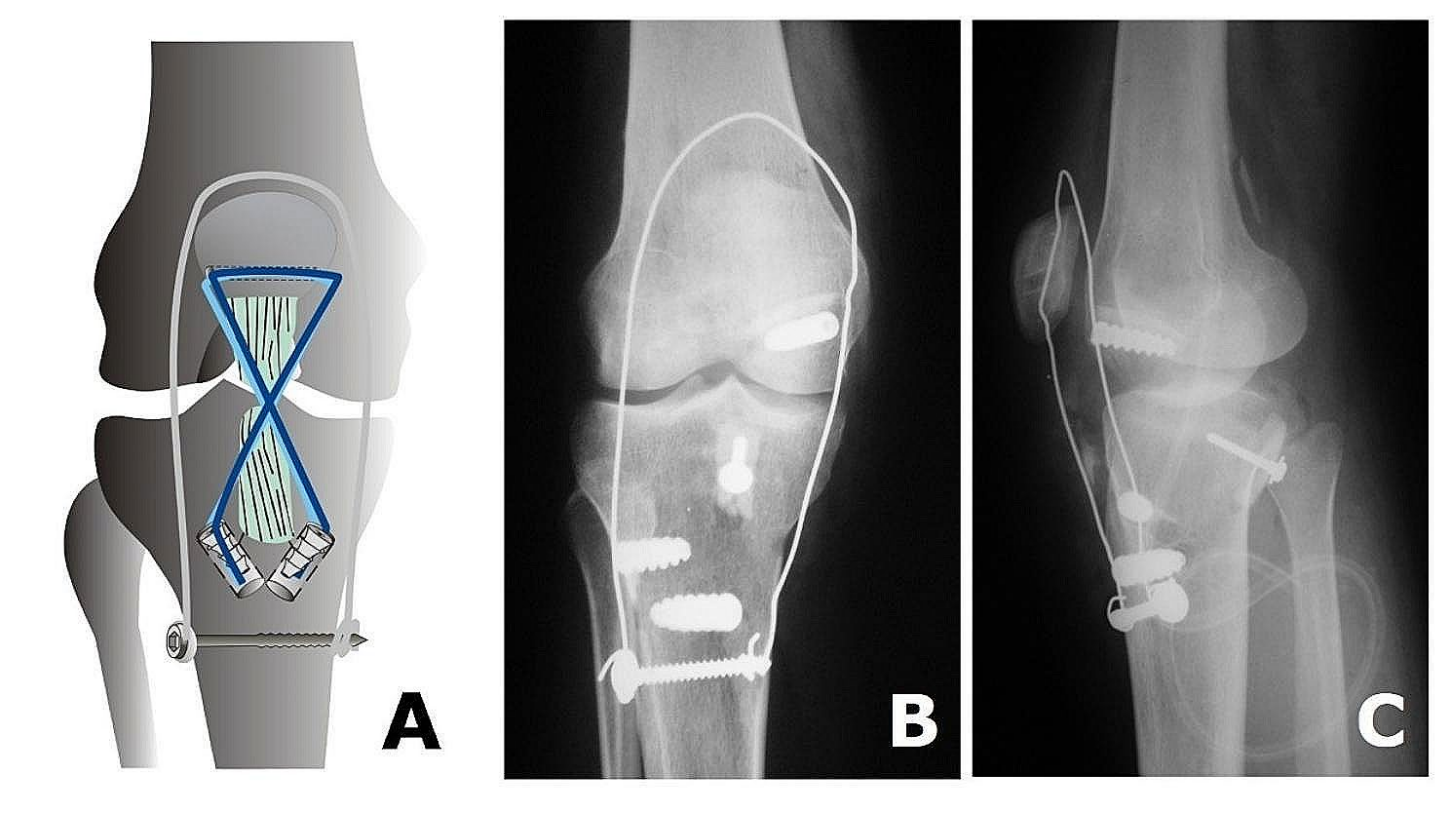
The patellar tendon and PCL reconstructions (A) and postoperative radiographs ((A) anteroposterior and lateral views (B))
